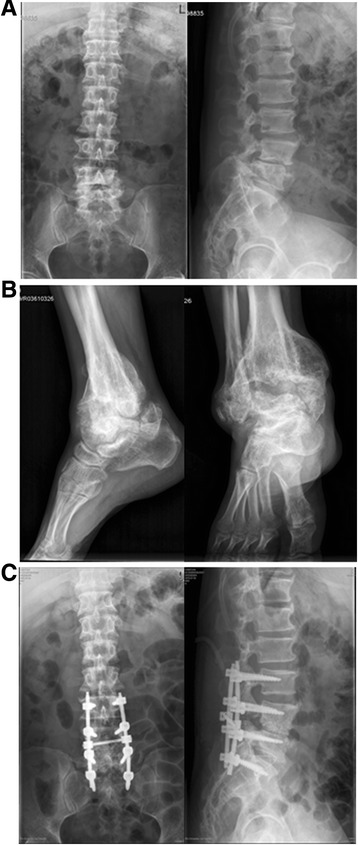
a. X-ray of the lumbar spine revealed narrowing of intervertebral space between L4 and L5. b. X-ray of left ankle showed severe destruction and disorganisation in a Charcot joint. c. Postoperative X-ray of lumbar spine showed well fixation and bone grafting
